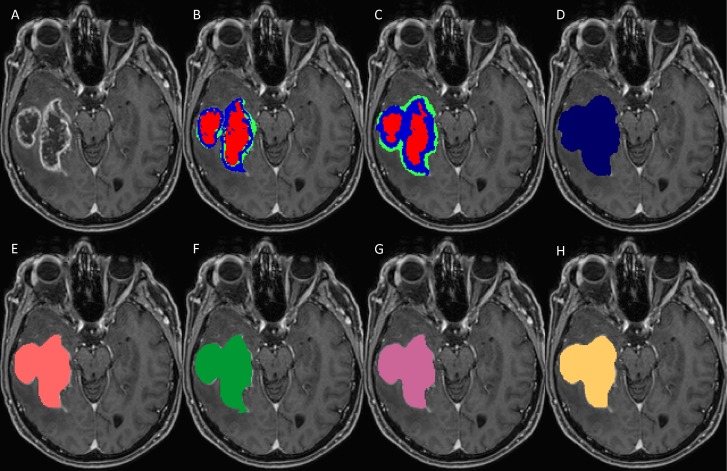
Set of MRI sequences used in this study for manual, automatic, and semi-automatic tumor volumetry. Original T1-weighted post-contrast MRI slice (A), manual subcompartmental segmentation into non-enhancing tumor (green), enhancing tumor (blue), and necrotic tissue (red) (B). BT subcompartmental segmentation into non-enhancing tumor (green), enhancing tumor (blue) and necrotic tissue (red) (C). BT core tumor segmentation (dark blue, D), SB1 core tumor segmentation (light red, E), SB2 core tumor segmentation (green, F), SB3 core tumor segmentation (purple, G) and SB4 core tumor segmentation (yellow, H).
